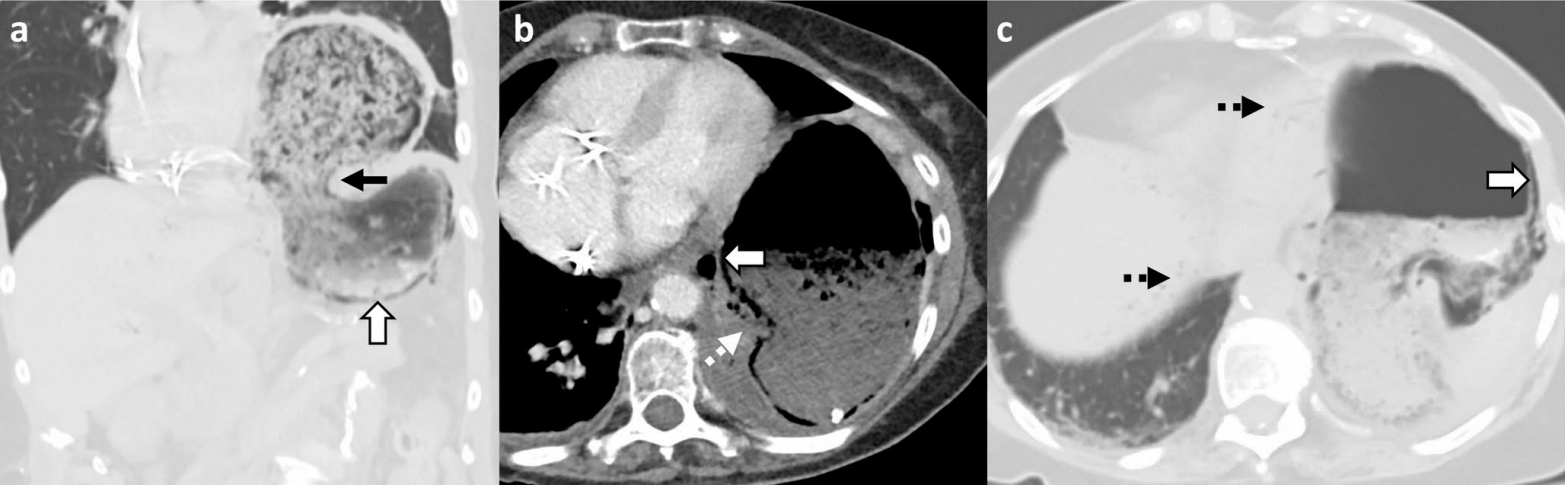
Emphysematous Gastritis. An 83-year-old diabetic patient with right upper quadrant pain and elevated serum lactate. CT images show gastric herniation with volvulus (black arrow, a), air in the gastric wall (white block arrow, a–c), gastric wall thickening (dotted white arrow, b), and portal venous gas (dotted black arrows, c). Conservative management was attempted due to advanced age. The patient died a day after the CT
